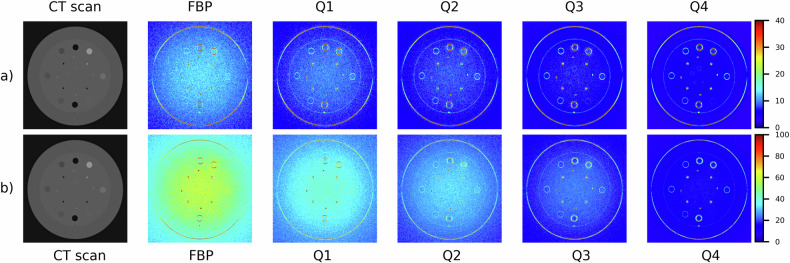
Photon-counting detector computed tomography scanner: noise (HU) maps of CT image of the CTP404 module reconstructed using both FBP and QIR algorithm with increasing iterative power (*i.e*., Q1, Q2, Q3, and Q4), for the smoother (a) and sharper (b) reconstruction kernel. For these reconstruction kernels, different colormap ranges are used to better show differences when varying iterative power*.* FBP, Filtered back projection; QIR, Quantum iterative reconstruction
